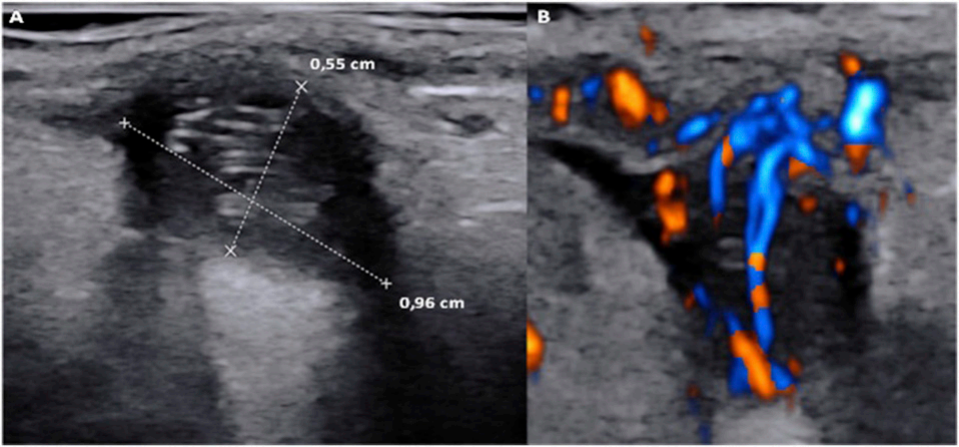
(A) A cystic lesion approximately of 1 cm in diameter, localized in the submucosa of the left superior maxillary vestibule, containing anechogenic fluid and a solid filiform mobile worm-like structure with echogenic posterior reinforcement (fluent weak movements were video recorded; see Supplemental Video 1). (B) Color doppler ultrasonography of the same cystic lesion showing suggestive twinkling artifact and no typical nor suspicious vascular pattern. This figure appears in color at www.ajtmh.org.
